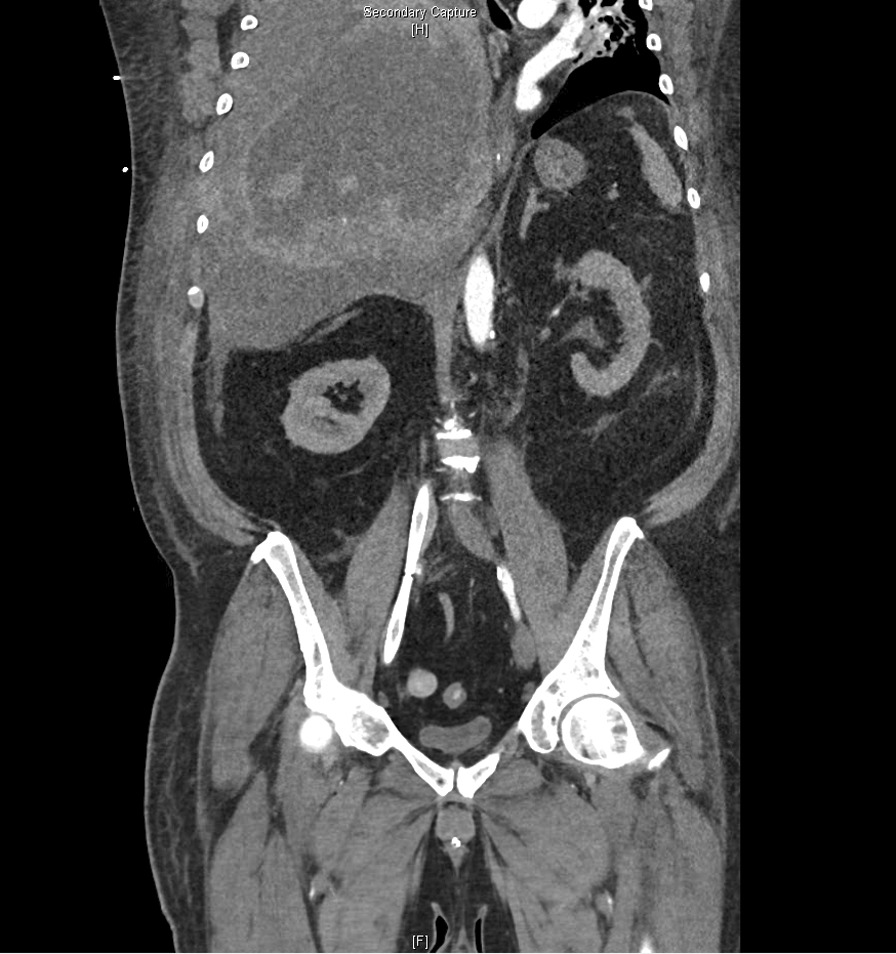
Coronal slice from multiplanar reformation computed tomography (Se:904, lm82). Phrenoptosis due to massive hemothorax of the right hemithorax
